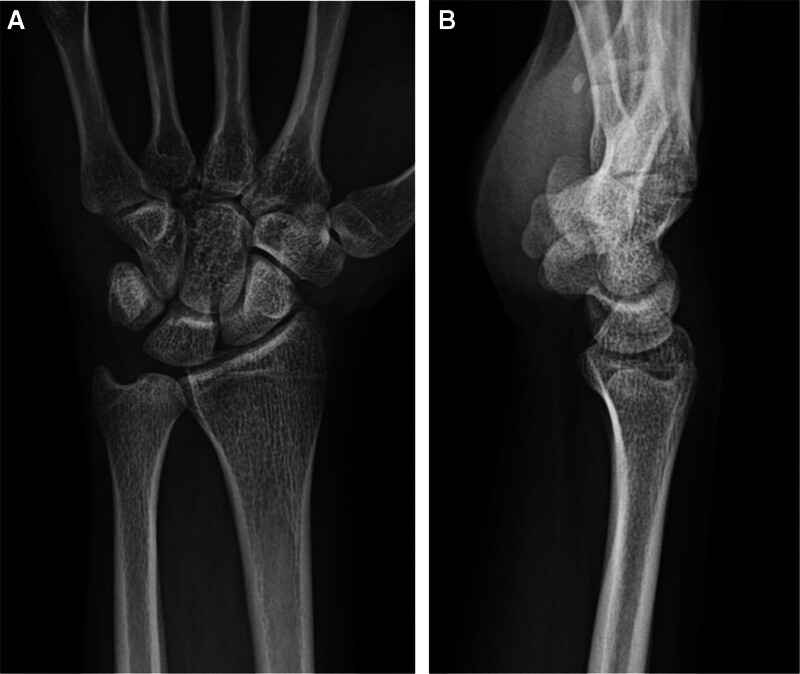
(A, B). Initial plain radiographs of the patient. Posterior-anterior (A) and lateral (B) View showed no abnormalities.
